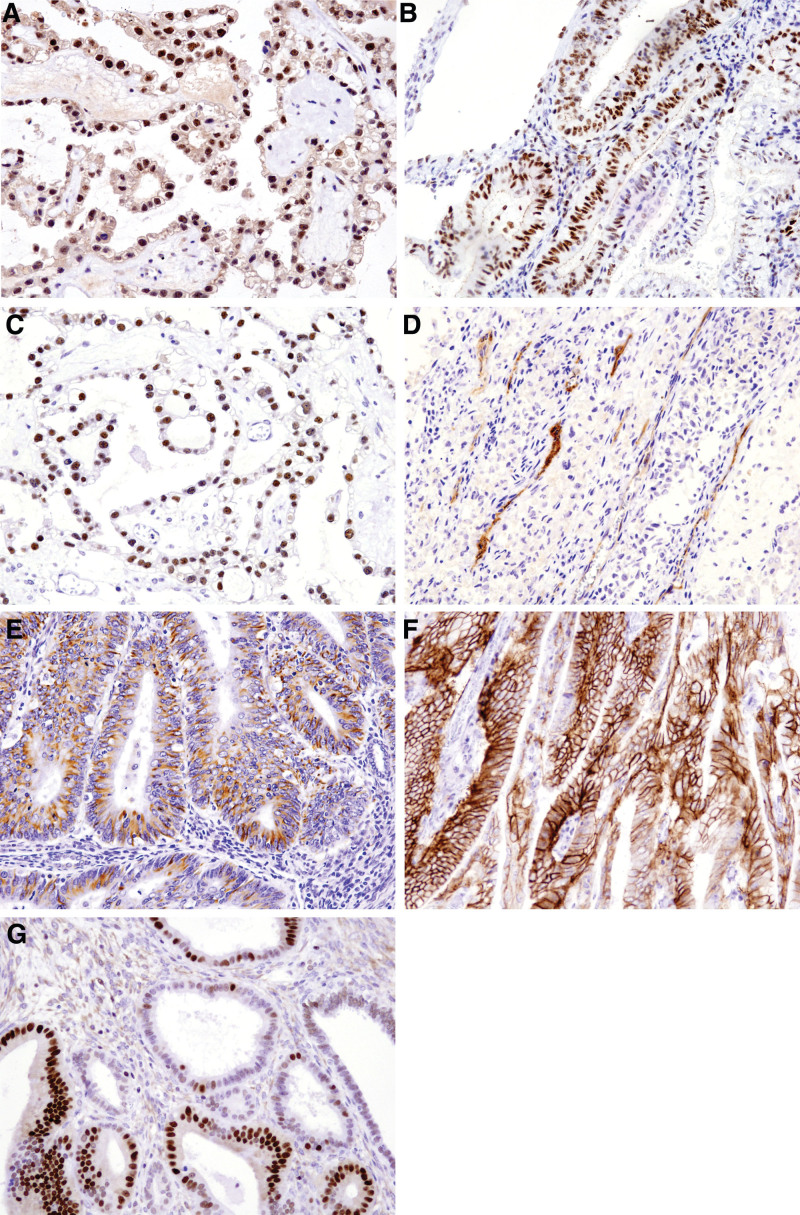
HIF-1α (×40) (A), STAT3 (×40) (B), and HNF-1β (×40) (C) expression in tumor nuclei. The neovascular cytoplasm of the tumor appears positive for PSMA (D) (×40). The tumor cytoplasm also appears positive for VIM (E) and AQP3 (F). FOXO1 (G) (×40) expression appears either absent or reduced in all cancers. AQP3 = aquaporin-3; FOXO1 = forkhead box O1; HIF-1α = hypoxia-inducible factor-1 subunit alpha; HNF-1β = hepatocyte nuclear factor-1 beta; PSMA = prostate-specific membrane antigen; STAT3 = signal transducer and activator of transcription 3; VIM = vimentin.
